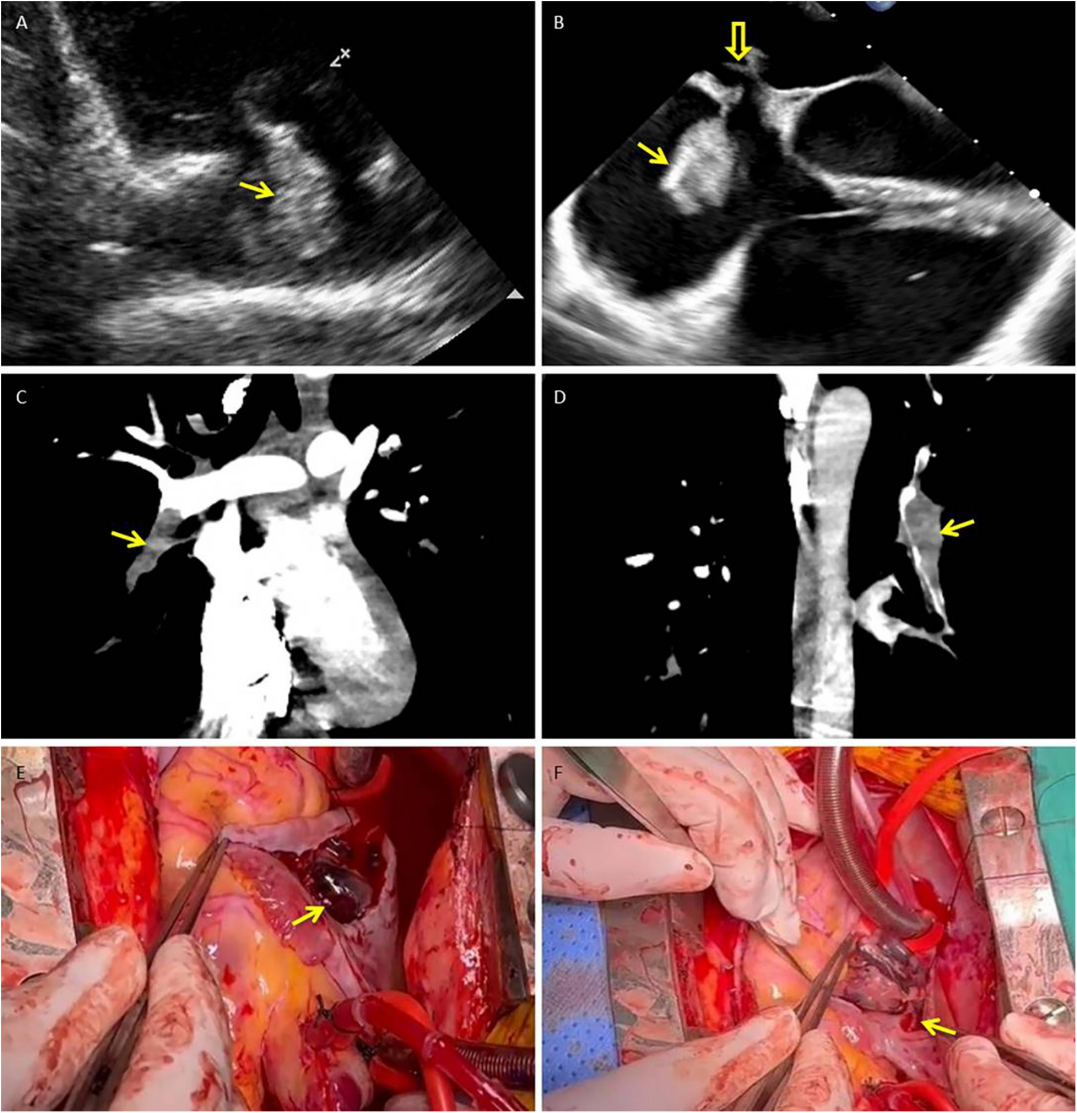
Thrombus in transit straddling a patent foramen ovale with concurrent pulmonary embolism: multimodality documentation and operative retrieval. (A) Transthoracic echocardiography (right ventricular inflow view) shows a highly mobile, serpiginous thrombus within the right atrium (arrow). (B) Transesophageal echocardiography (mid-esophageal four-chamber view) demonstrates the thrombus straddling the PFO (solid arrow), with its distal segment prolapsing into the left atrium (hollow arrow); right-sided chamber enlargement is present. (C,D) CT pulmonary angiography (coronal reformations) reveals acute pulmonary emboli, including an intraluminal filling defect in the right lower-lobe pulmonary artery (C) and additional defects in distal branches of the left pulmonary artery (D) (arrows). (E,F) Intraoperative photographs: (E) shows the large, friable thrombus (measuring approximately 2.0 × 8.5 cm) immediately after the right atrium was opened (arrow). (F) demonstrates the narrow stalk (approximately 3 mm) of the thrombus arising from the PFO ostium (arrow). PFO, patent foramen ovale; RA, right atrium; LA, left atrium; RV, right ventricle; TTE, transthoracic echocardiography; TEE, transesophageal echocardiography; CTPA, CT pulmonary angiography.
